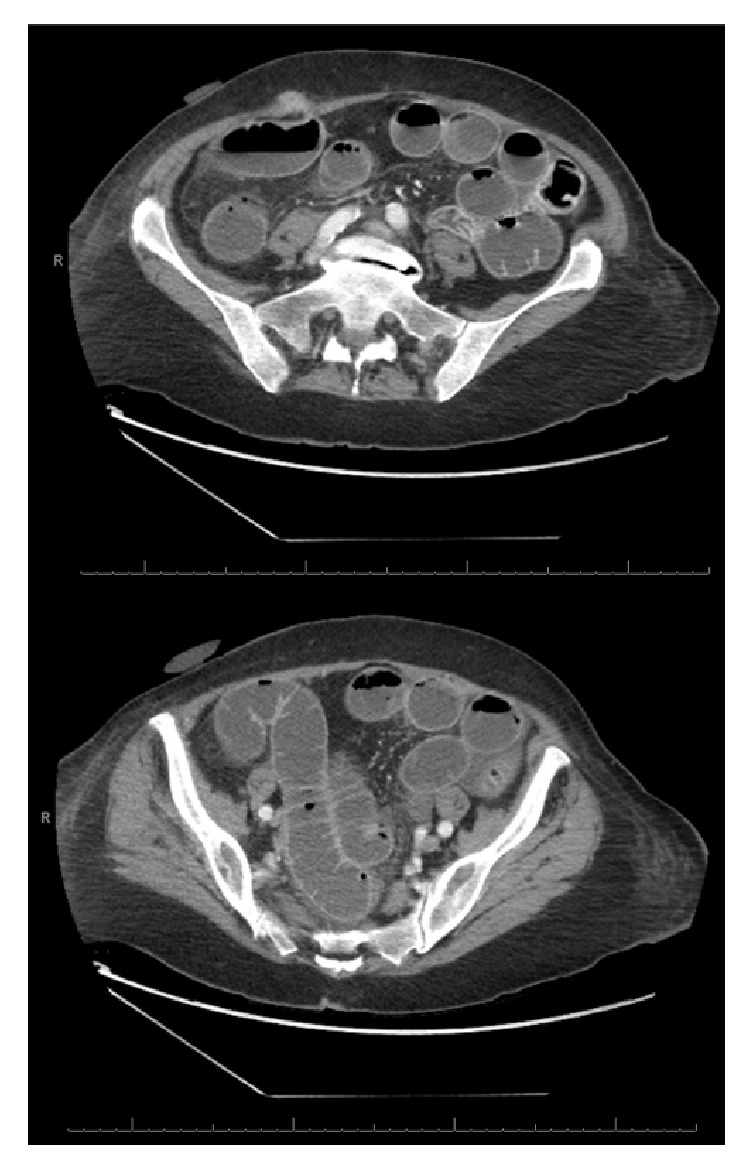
First CT scan abdomen and pelvis. Contrast-enhanced axial images showing port site hernia with diffuse small bowel dilation.
